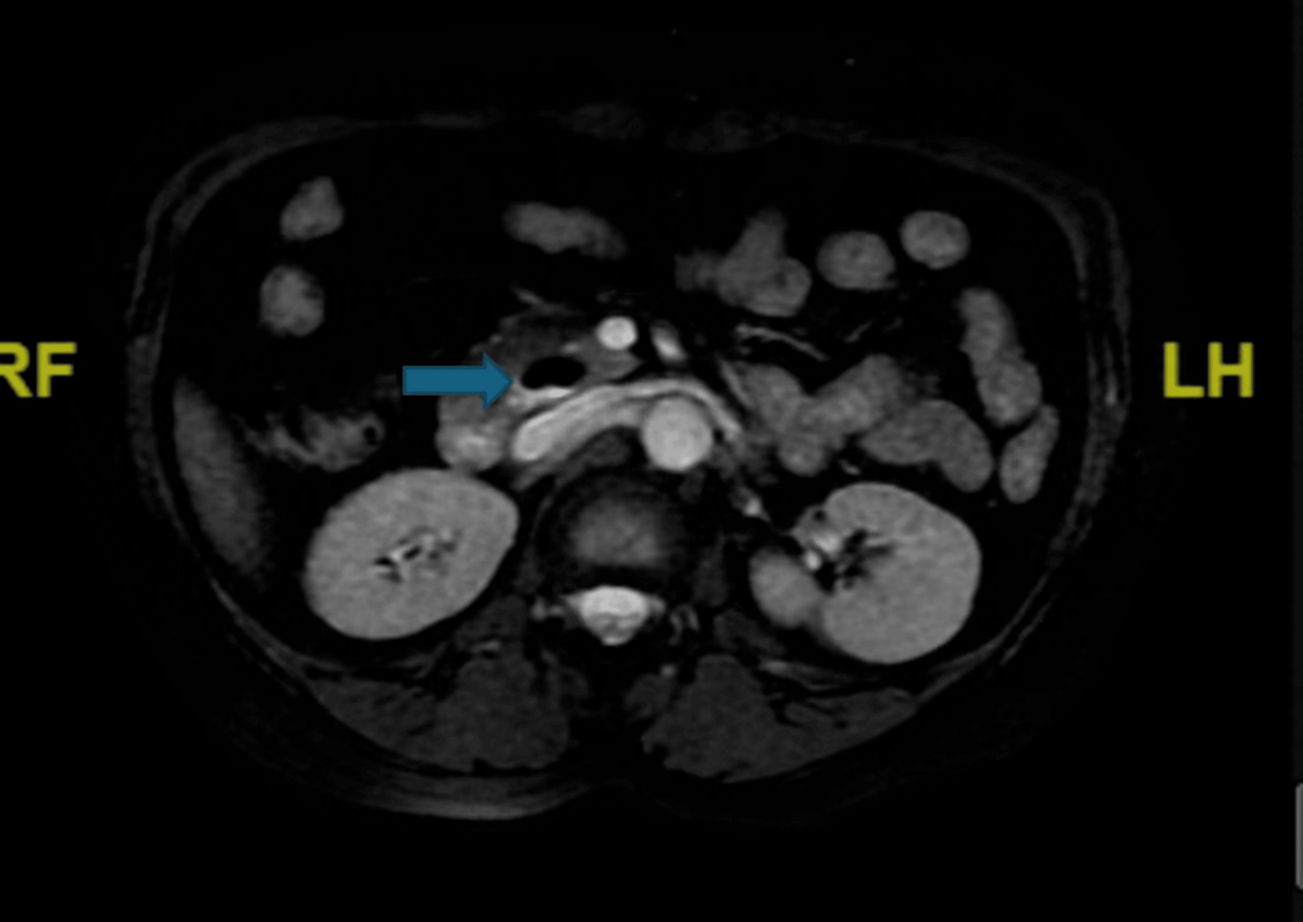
Duodenal diverticulitis identified on magnetic resonance imaging Axial T2-weighted magnetic resonance imaging (MRI) of the abdomen, performed as a follow-up study, reveals a small, well-circumscribed, fluid-filled outpouching arising from the second portion of the duodenum, consistent with a duodenal diverticulum (arrow). Surrounding high-signal intensity indicates associated inflammation (diverticulitis), establishing it as the definitive underlying cause of the patient's recurrent acute pancreatitis.
